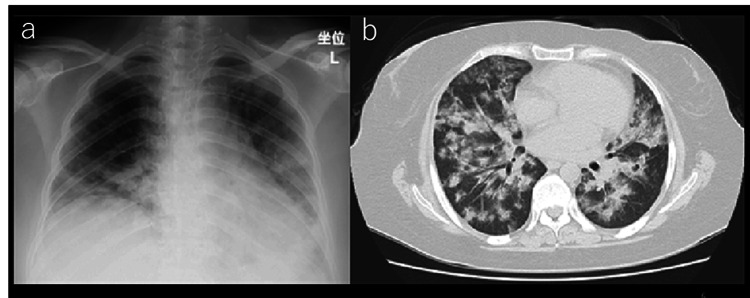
Chest radiography (a) and CT scan (b) following each occurrence of DAH DAH: Diffuse alveolar hemorrhage
